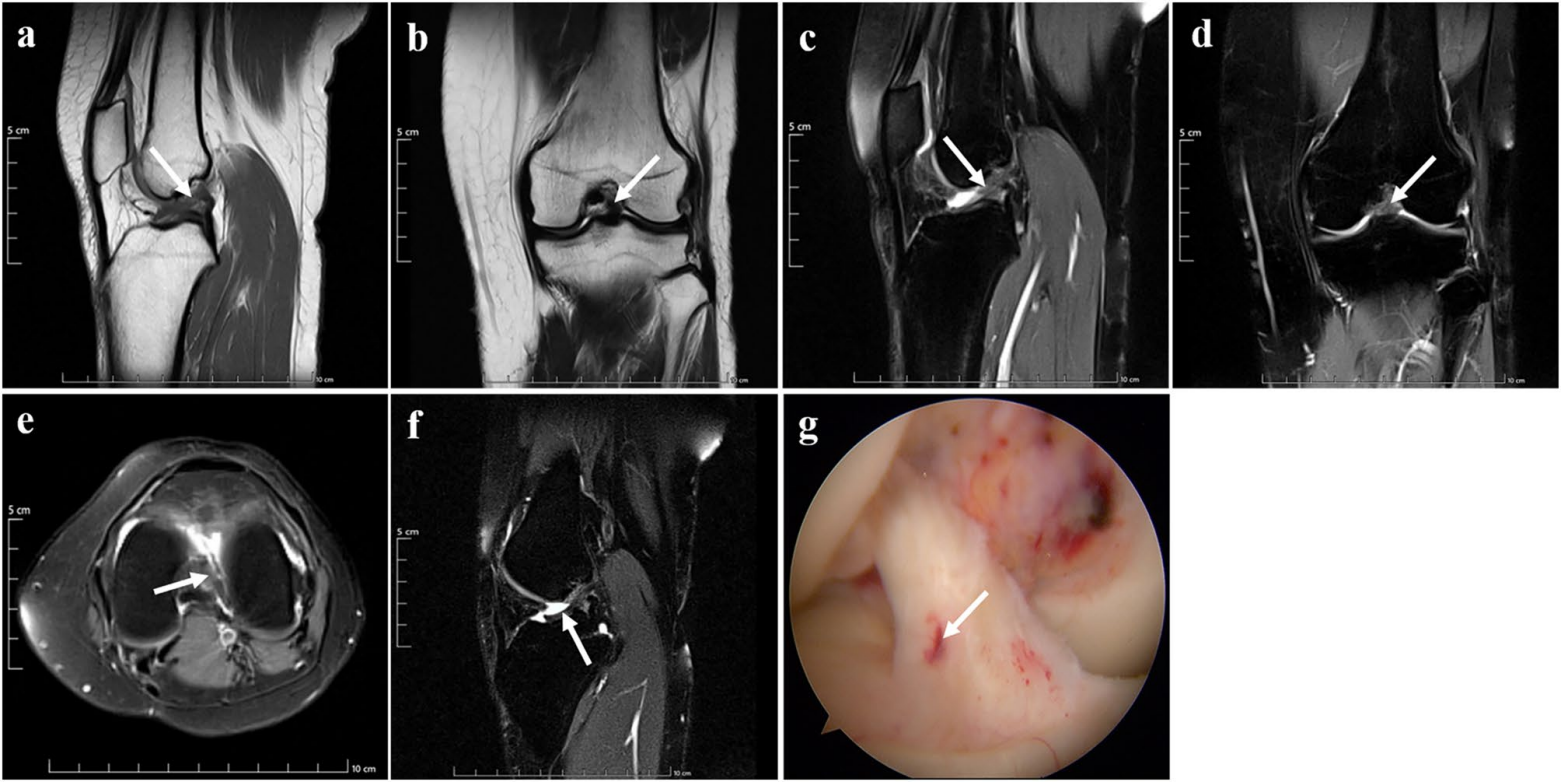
Grade I injury of ACL presence on MRI and arthroscopy of a 34-years-old woman. The shape and signal intensity of the ACL (arrows) was similar to the normal ligament on sagittal T_1_WI (a), coronal T_2_WI (b), Sagittal PDWI (c), coronal PDWI (d), and axial PDWI (e) with effusion of knee joint on PDWI. The ligament was tortuous as “*S*-shaped” and localized edema with increased signal intensity in the tibial part on the new modified DPP-TSO-Sag-FS-PDWI (arrow on (f)). Arthroscopy showed the ligament was loose with a hematoma in the anterior tibial part of the ligament (arrow on (g)).
